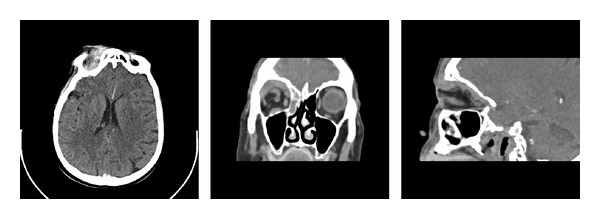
Triplanar views of a noncontrast CT scan showing opacification of the right ethmoidal and frontal sinuses with medial orbital extension.
